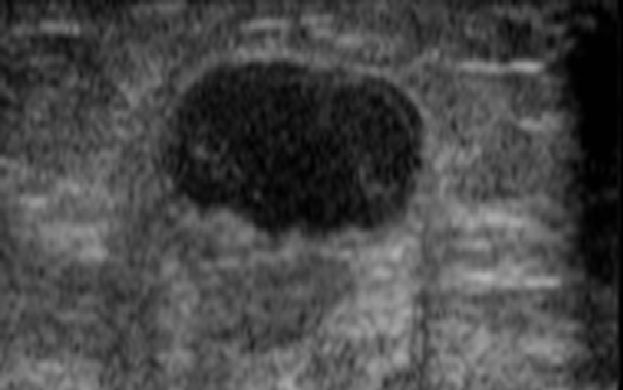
Ultrasound image (bitmap format) showing hypoechoic malignant breast lesion.
